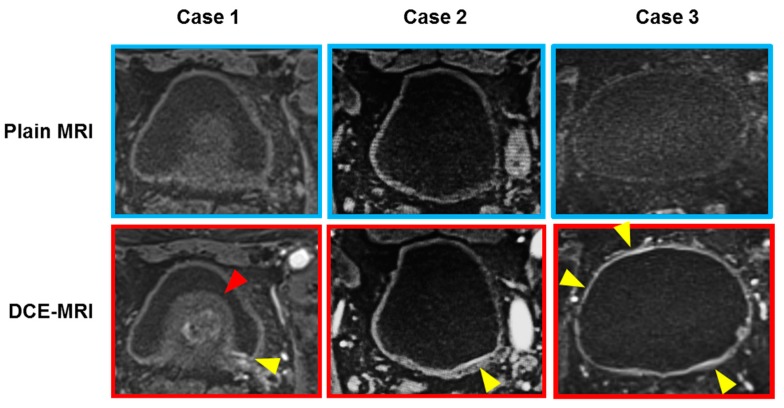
Three representative cases with flat lesions suspected bladder carcinoma in situ (CIS). Heterogeneous and blurry contrast-enhancement of the bladder mucosa and the submucosa (lamina propria) at the arterial phase of DCE-MRI is considered as a case of bladder CIS (yellow arrowheads). Case 1: A 71-year-old man with T4 high-grade urothelial carcinoma with CIS. DCE-MRI shows submucosal enhancement around the left ureteral orifice, which was pathologically proven CIS. The suspected CIS lesion (yellow arrowhead) was difficult to look at with the cystoscopy because of the enlarged benign prostate (red arrowhead). Case 2: An 88-year-old man with an isolated CIS on the posterior wall. Case 3: A 70-year-old woman with broad-range CIS in the whole bladder wall.
